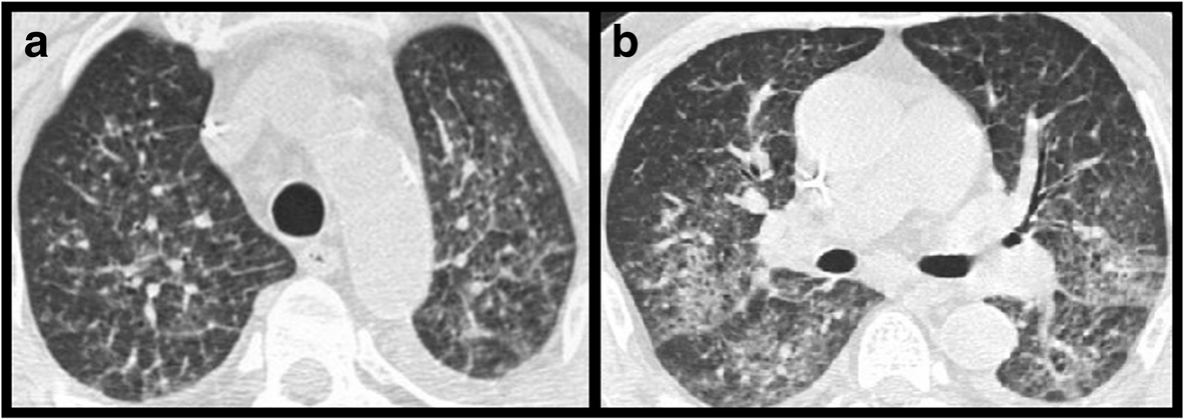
Viral pneumonia from cytomegaly virus. Patient with chemotherapy for Non-Hodgkin Lymphoma. a, b Chest CT at the level of the aortic arch (a) and the pulmonary trunk (b) at lung windows. Bilateral reticular-nodular interstitial pattern, consolidation and ground glass
